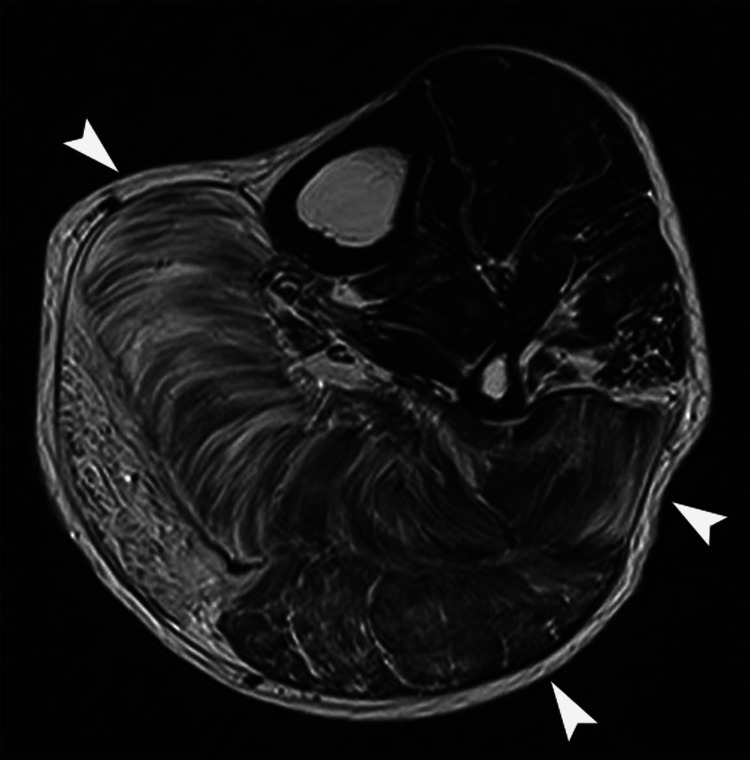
A patient with hereditary advanced limb-girdle muscular dystrophy and neuropathy. Axial T2-weighted MR image of the calf shows diffuse abnormal high signal intensity in enlarged soleus and gastrocnemius muscles (arrowheads), reflecting gross fatty replacement.
